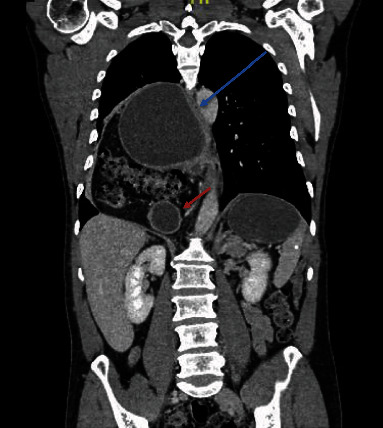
Computed tomography of the thorax, abdomen, and pelvis. Coronal reconstruction showing a giant HH involving the stomach and part of the duodenum and transverse colon. The blue arrow corresponds to the esophagus compressed by the hiatal hernia; the red arrow corresponds to the duodenum.
